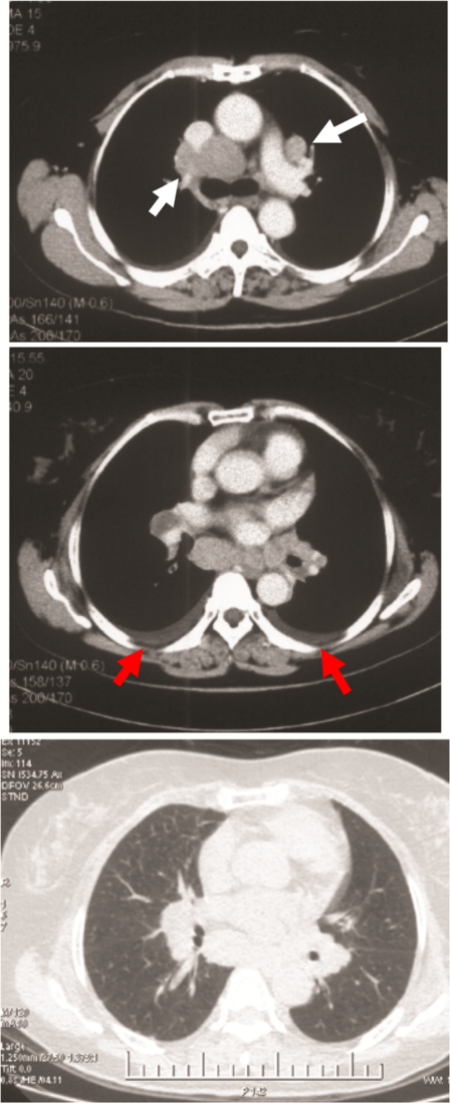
CT scans of the chest. An enhanced chest CT scans indicated a right hilar mass and multiple lymph node enlargements in the mediastinal and bilateral hilar areas of the lungs (*white arrows*), as well as bilateral small pleural effusions (*red arrows*)
